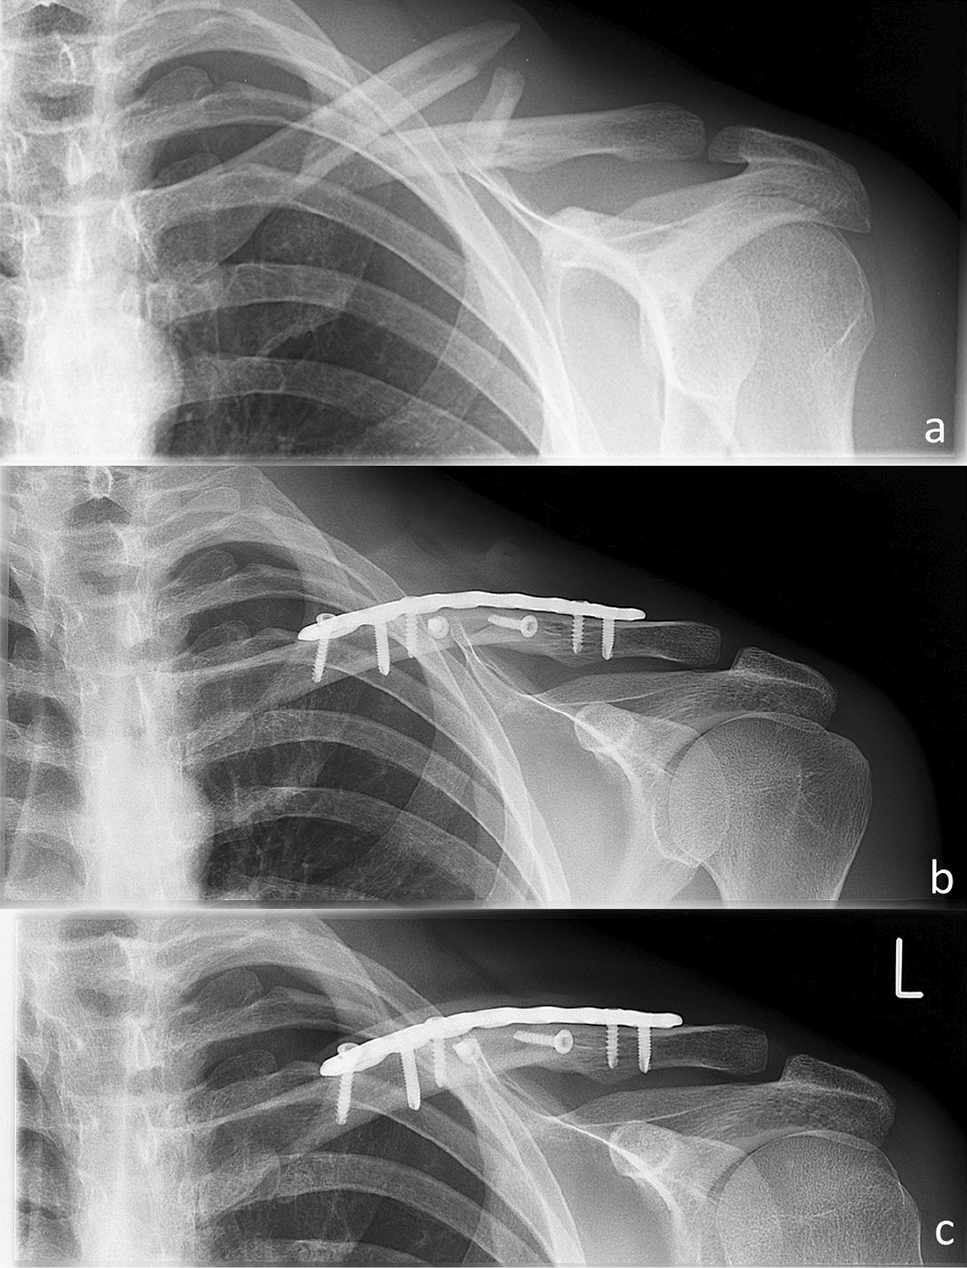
Preoperative (a) and postoperative (b) X-rays until fracture consolidation (c) of a fragmentary (OTA 2.B) midshaft clavicle fracture treated using 3.5-mm cortical screw augmentation and locking compression plate
